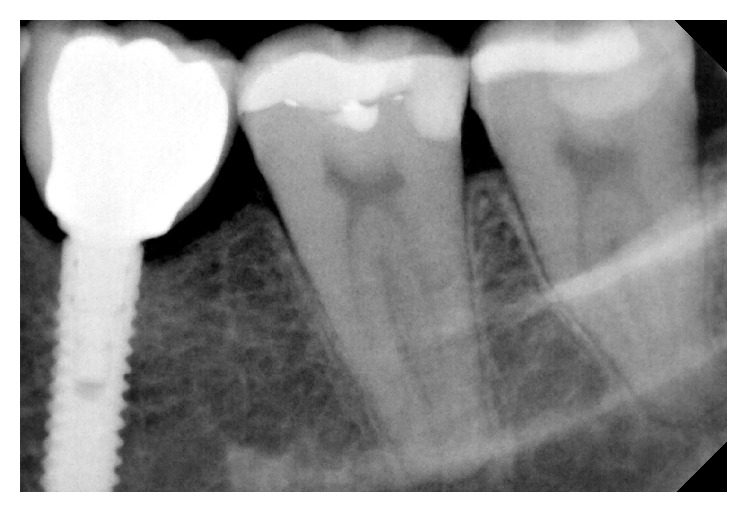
Periapical X-ray 1 year after loading.
